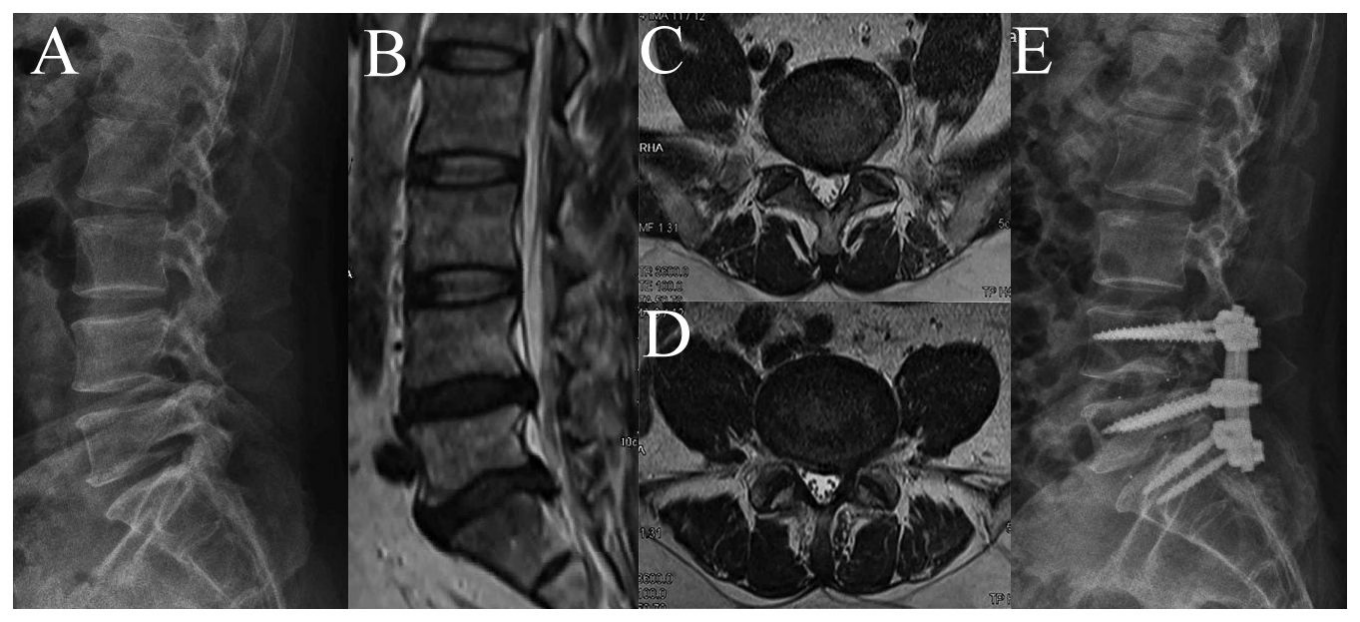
A 54-years-old man, diagnosed as LDH and left foot drop. (A) Preoperative radiography. (B) Preoperative mid-sagittal MRI showed LDH on L4-S1. (C) Preoperative axial MRI of L4-5 showed the left L5 nerve root compression. (D) Preoperative axial MRI of L5-S1 showed the left S1 nerve root compression. (E) Postoperative radiography.
